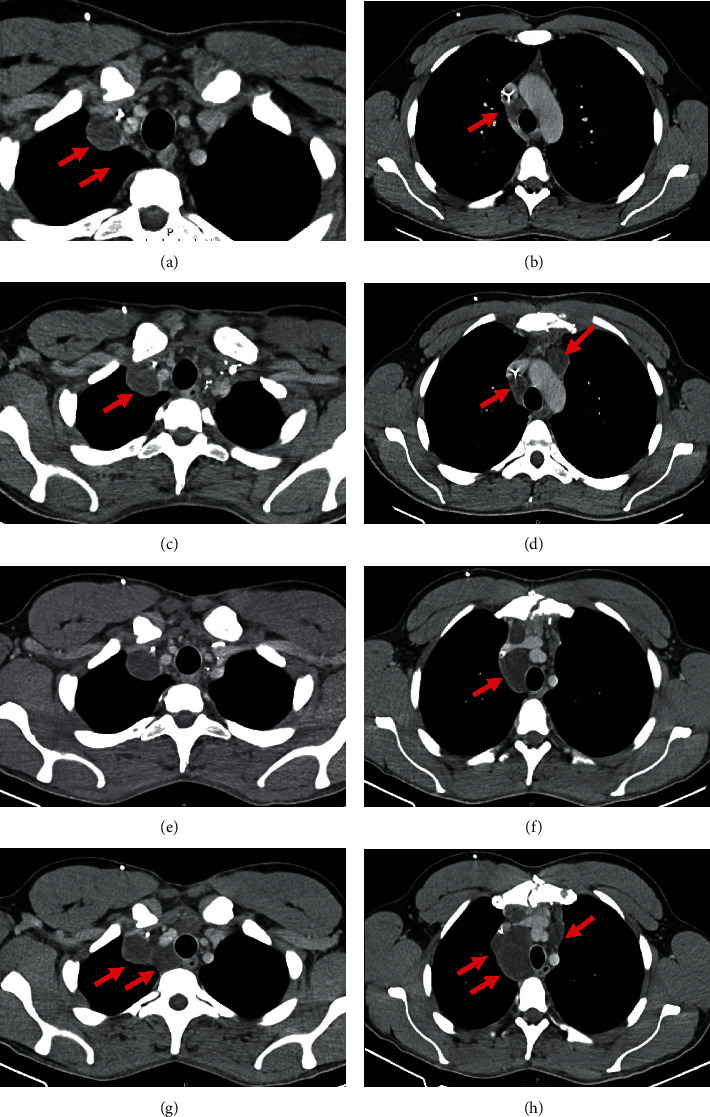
CT images. Axial thoracic contrast-enhanced CT images on two different mediastinal levels at 5-month (a, c), 7-month (c, d), 12-month (e, f), and 24-month (g, h) follow-up, depicting enlarging hypodense lymph nodes.
